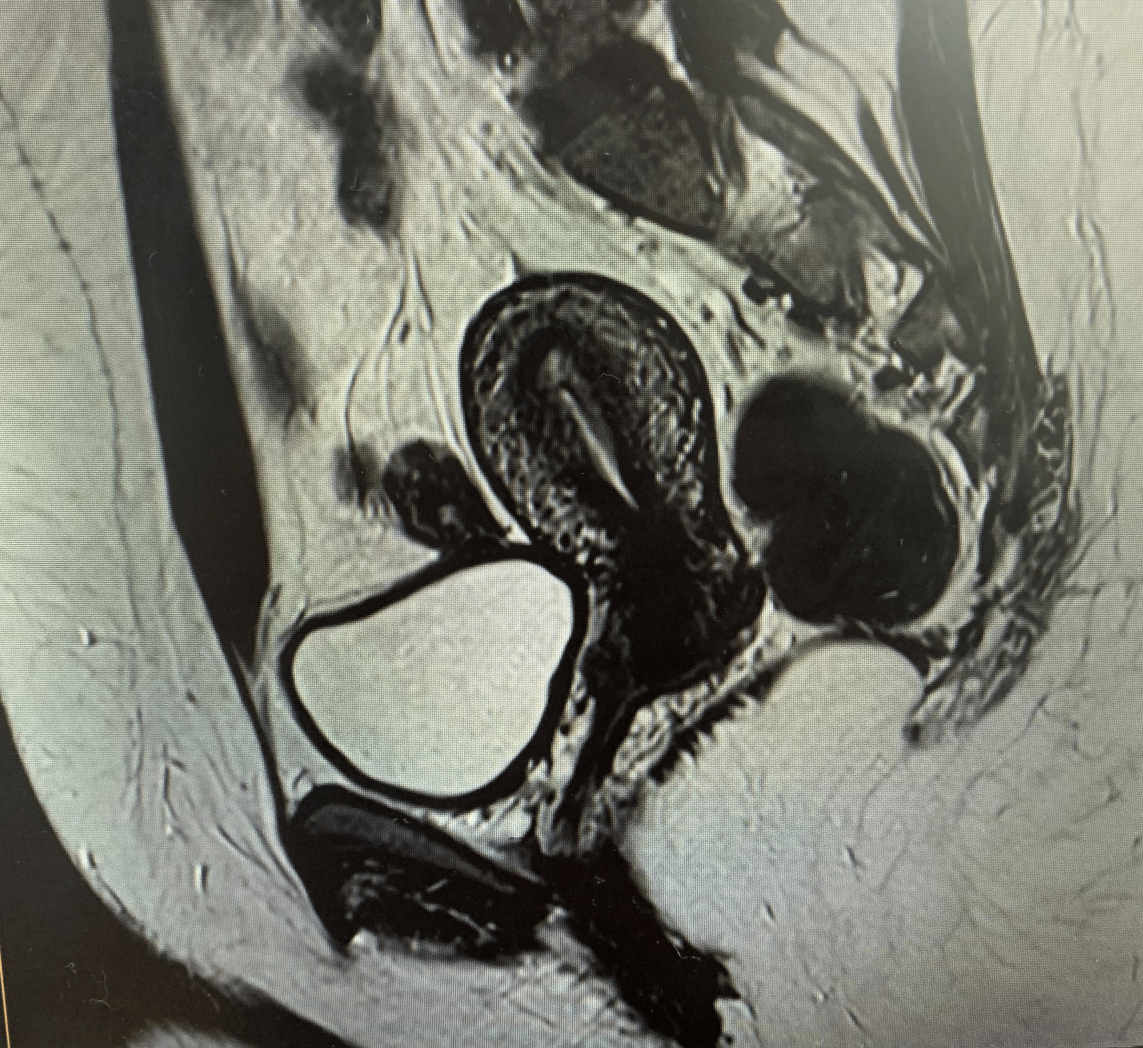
Postoperative MRI sagittal pelvic imaging MRI: Magnetic resonance imaging
